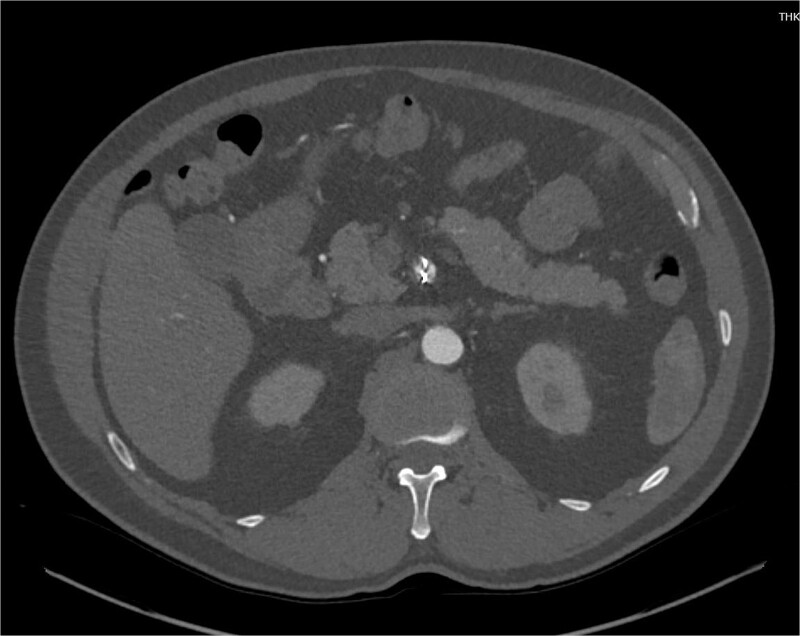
CTA demonstrating interval embolization of SMA at site of prior thrombus and dissection.
